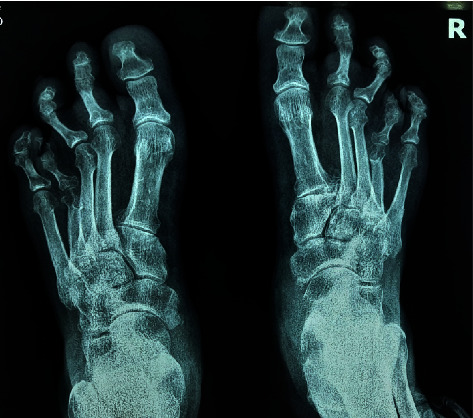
X-ray of bilateral feet showing brachymetatarsia.
